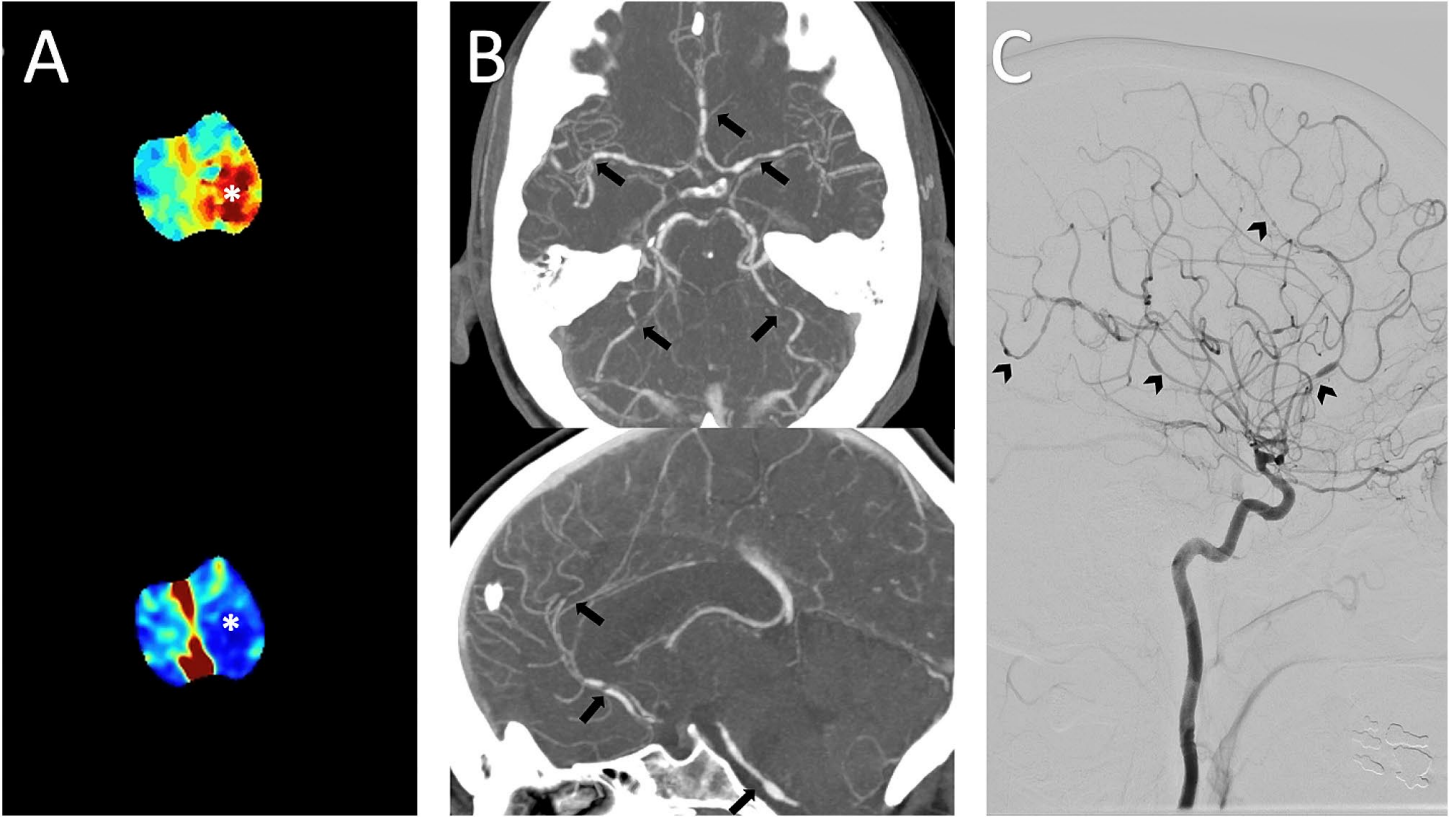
Initial imaging A: Axial CT perfusion Tmax (top) and cerebral blood flow (bottom) slices at the vertex demonstrated left-sided acute ischaemic penumbra and infarct core (*) B: CT angiogram maximum intensity projections (MIPs) axial (top) and sagittal (bottom) showed bilateral multifocal stenoses and beading (arrows) of the anterior and posterior circulations C: Interventional angiography of the left internal carotid artery sagittal projection confirmed similar findings (arrow heads)
